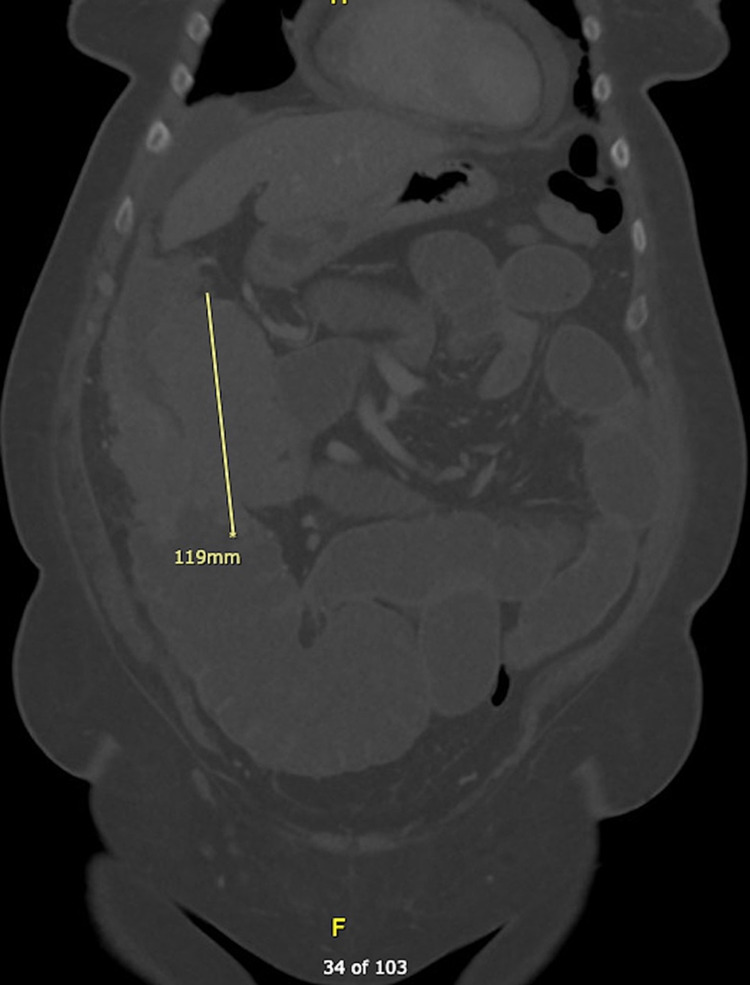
Computed tomography, coronal view, revealing an irregular mass at the mid to distal ascending colon with signs of obstruction.
